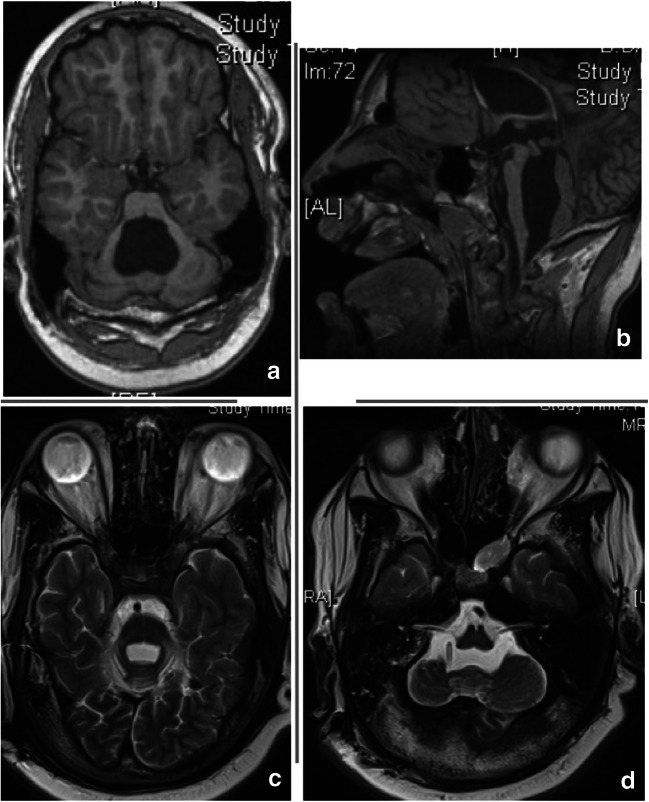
MR images demonstrating a TFV in a preterm with PHH, came at age of 15 years with fatigue and somnolence. a, b Axial and sagittal MR T1-weighted images showing ballooning of the fourth ventricle with brainstem compression. c, d Axial T2-weighted images 5 years after insertion of the fourth ventricular catheter and connection to implanted VP shunt
